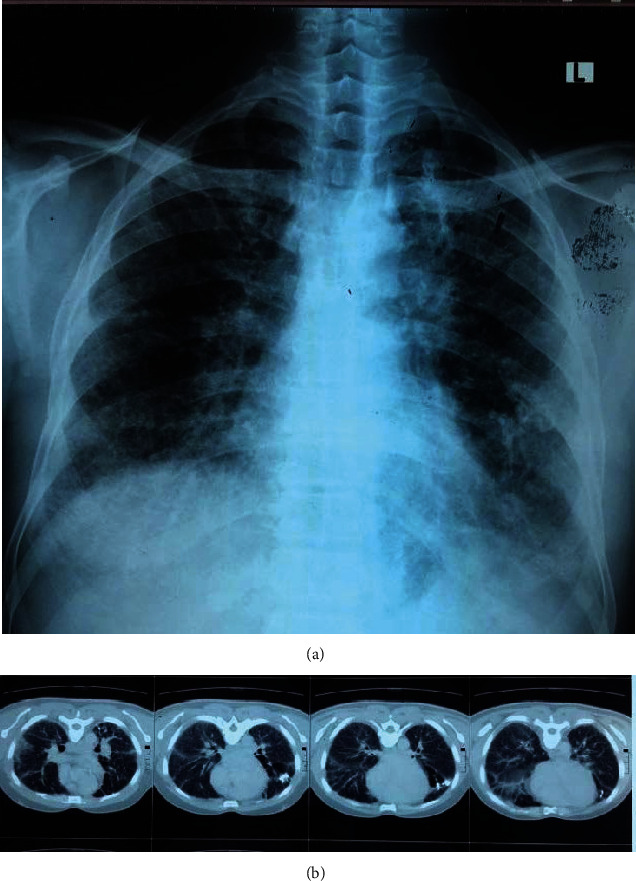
(a) Chest X-ray showing diffuse bilateral reticulonodular changes. (b) Chest CT scan showing diffuse bilateral reticulonodular opacities with multiple left lung calcifications and cardiomegaly with dilated pulmonary trunk.
